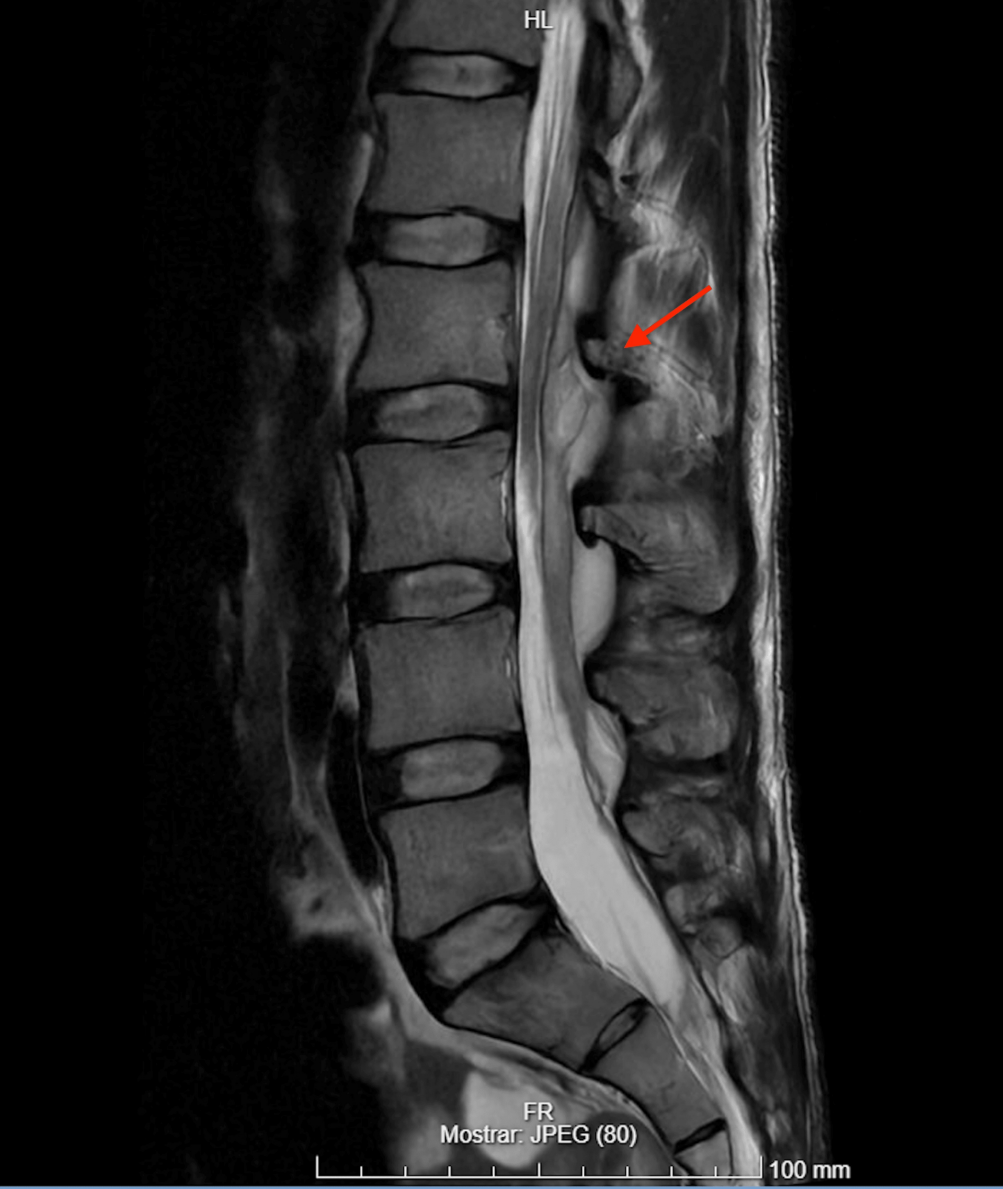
MRI revealing an epidural abscess (red arrow) extending from L1 to L4 with paravertebral involvement at the L2-L3 level MRI: magnetic resonance imaging
